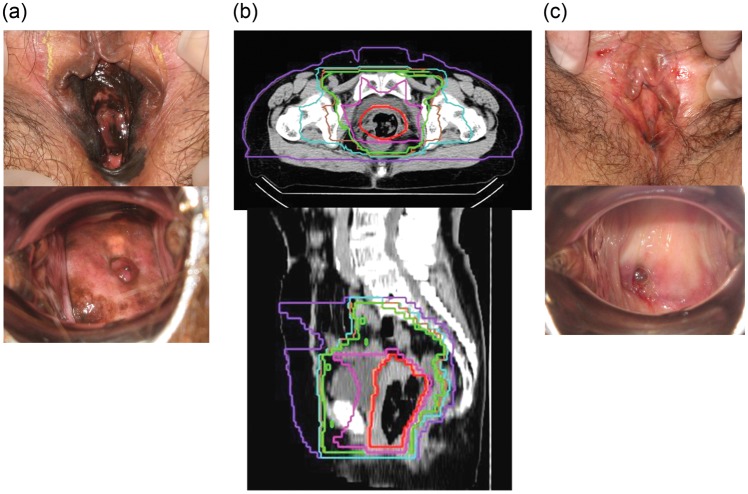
A case of T3N0M0 vulval MM treated with C-ion RT. (a) Genital examination findings before treatment. (b) Radiation field and dose distribution on axial and sagittal CT images. (c) One year after the treatment. The patient had irradiated 36 GyE to small pelvis and inguinal region, then reduced irradiation field to the tumor site up to a total of 67.6 GyE. Isodose curves are 96% (red), 90% (orange), 70% (pink), 60% (yellow green), 50% (green), 30% (cyan) and 10% (purple) of 57.6 GyE. She has been living without recurrence or adverse effect for 2 years.
